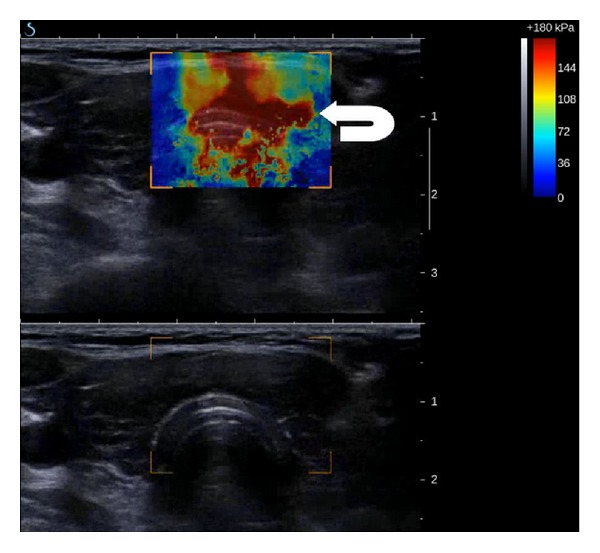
An artifact (arrow) from tissue compression on elastogram (upper image) in patient with Hashimoto thyroiditis, with no real focal lesions evident on B-mode ultrasound (lower image). A hard stiff pseudolesion is generated (see also Supplementary material 1 available online on doi: 10.1155/2012/657147). To avoid such artifacts no pressure on the probe during elasticity evaluation should be applied.
